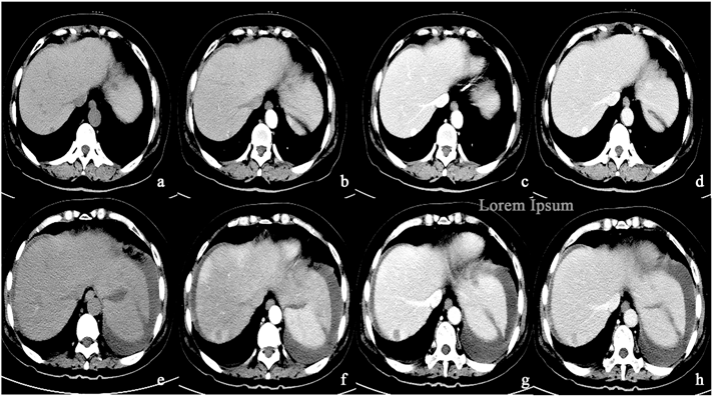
(A–D) A round low-density mass in the right lobe of the liver was shown in the plain CT. The lesions demonstrate peripheral nodular discontinuous enhancement in the arterial phase and centripetal filling in the portal and delayed phases. (E–H) After treatment with Bevacizumab, the lesions showed circular enhancement.
